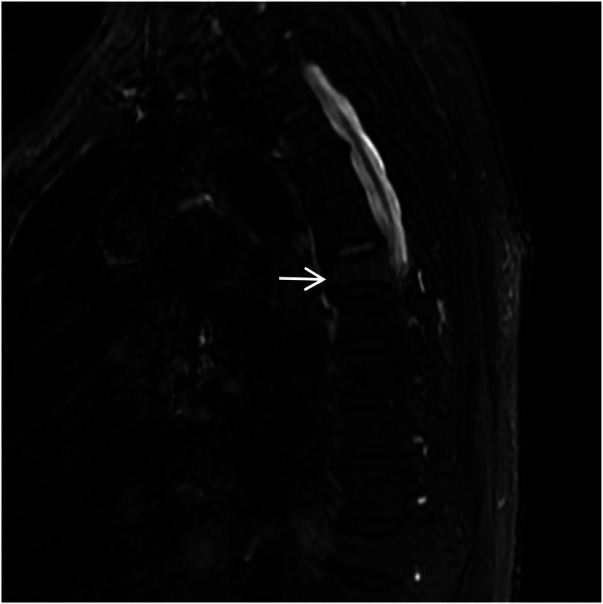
Sagittal STIR MRI of the thoracic spine shows high signal within the T6 (white arrow) vertebral body, with patchy high signal shadows in the soft tissues of the back at the same level.
